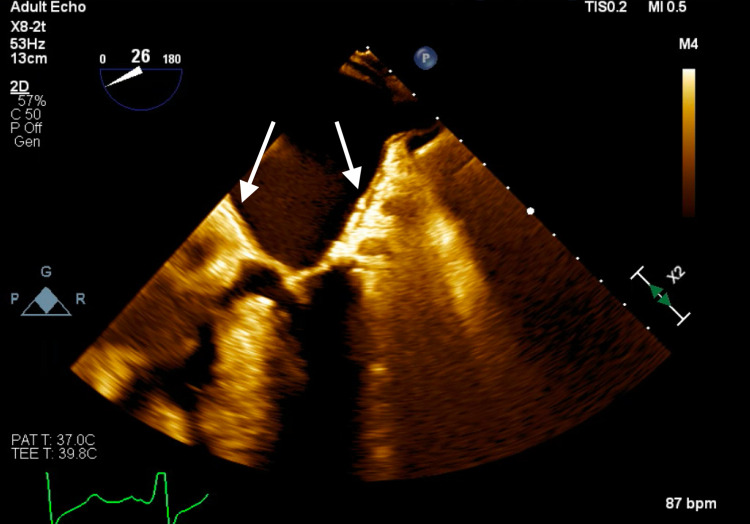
Transesophageal echocardiogram four-chamber view showing layering of highly echogenic material along the posterior left atrial wall.
